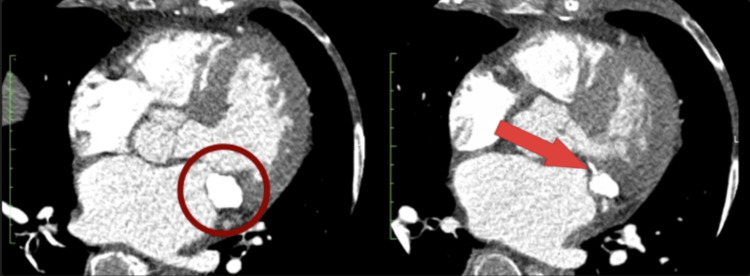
Imaging with CT revealed a large, calcified vegetation on the posterior aspect of the mitral annulus. Also shown is its proximity to the left circumflex artery CT: computed tomography
